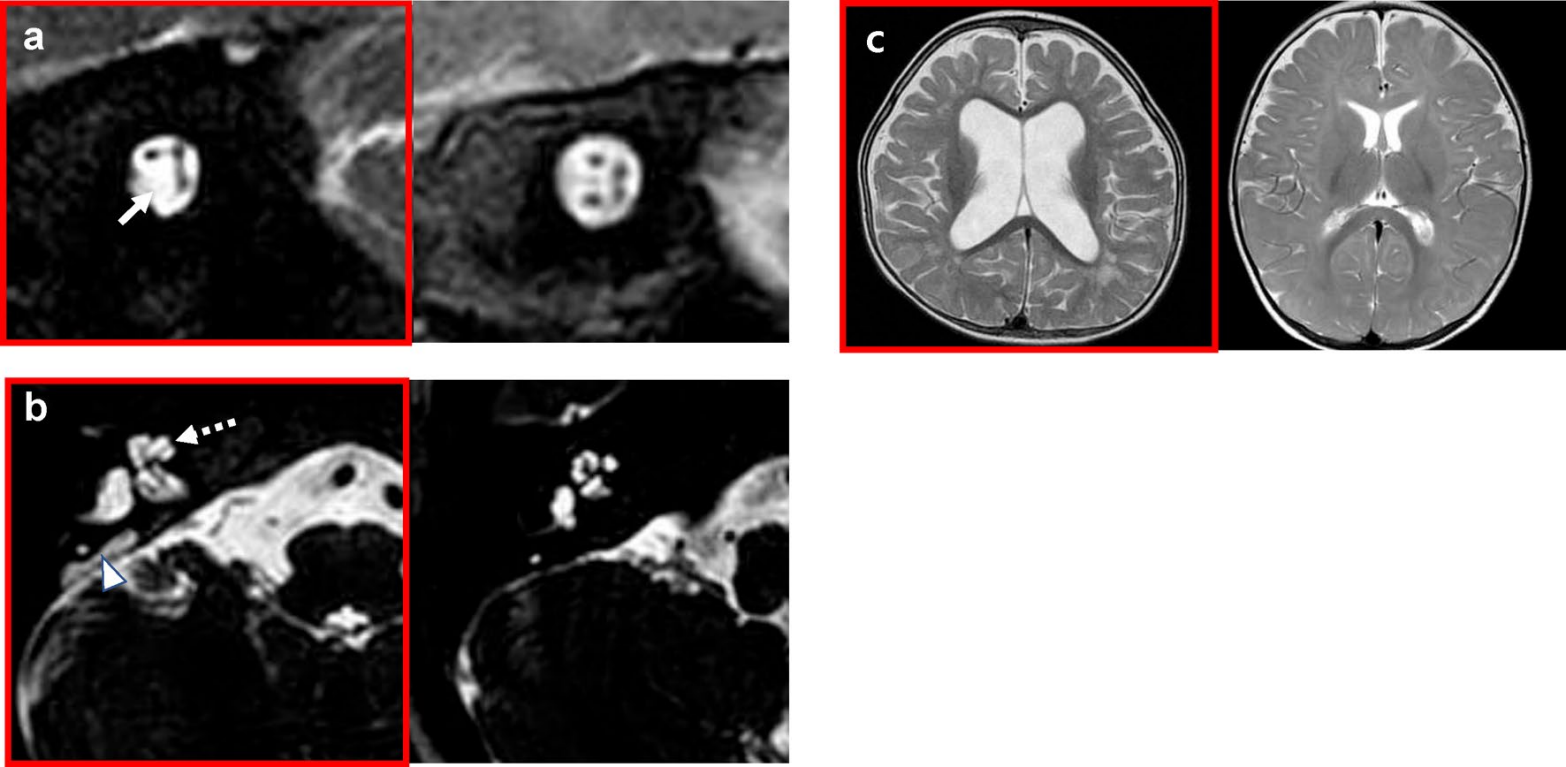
Three major abnormalities related to pediatric SP-SNHL as seen in IAC-MRI. Images on the left side show abnormal findings, while normal findings are on the right. (a) Cochlear nerve deficiency: Cochlear nerve is not observed in the internal auditory canal (arrow). (b) Enlarged vestibular aqueduct with incomplete partition type 2: Vestibular aqueduct is enlarged and conical in shape (arrowhead), and the interscalar septum is not present (dotted arrow). (c) Periventricular leukomalacia: Ventriculomegaly with irregular margins of the bodies of the lateral ventricles and loss of periventricular white matter are observed.
